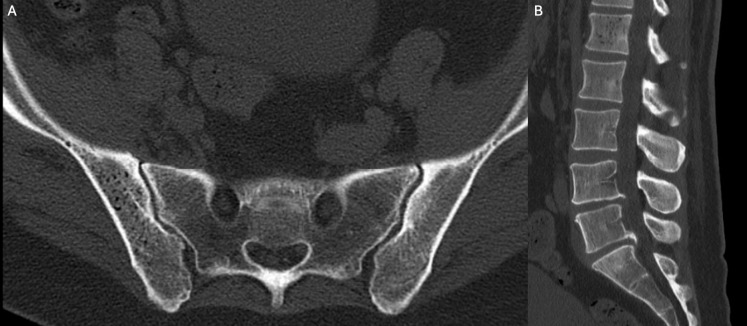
Axial CT of the pelvis (A) and sagittal CT reformation of the lumbar spine (B) demonstrate multiple low-attenuation foci in keeping with intraosseous gas within the right iliac wing and the L1 vertebral body, respectively.
